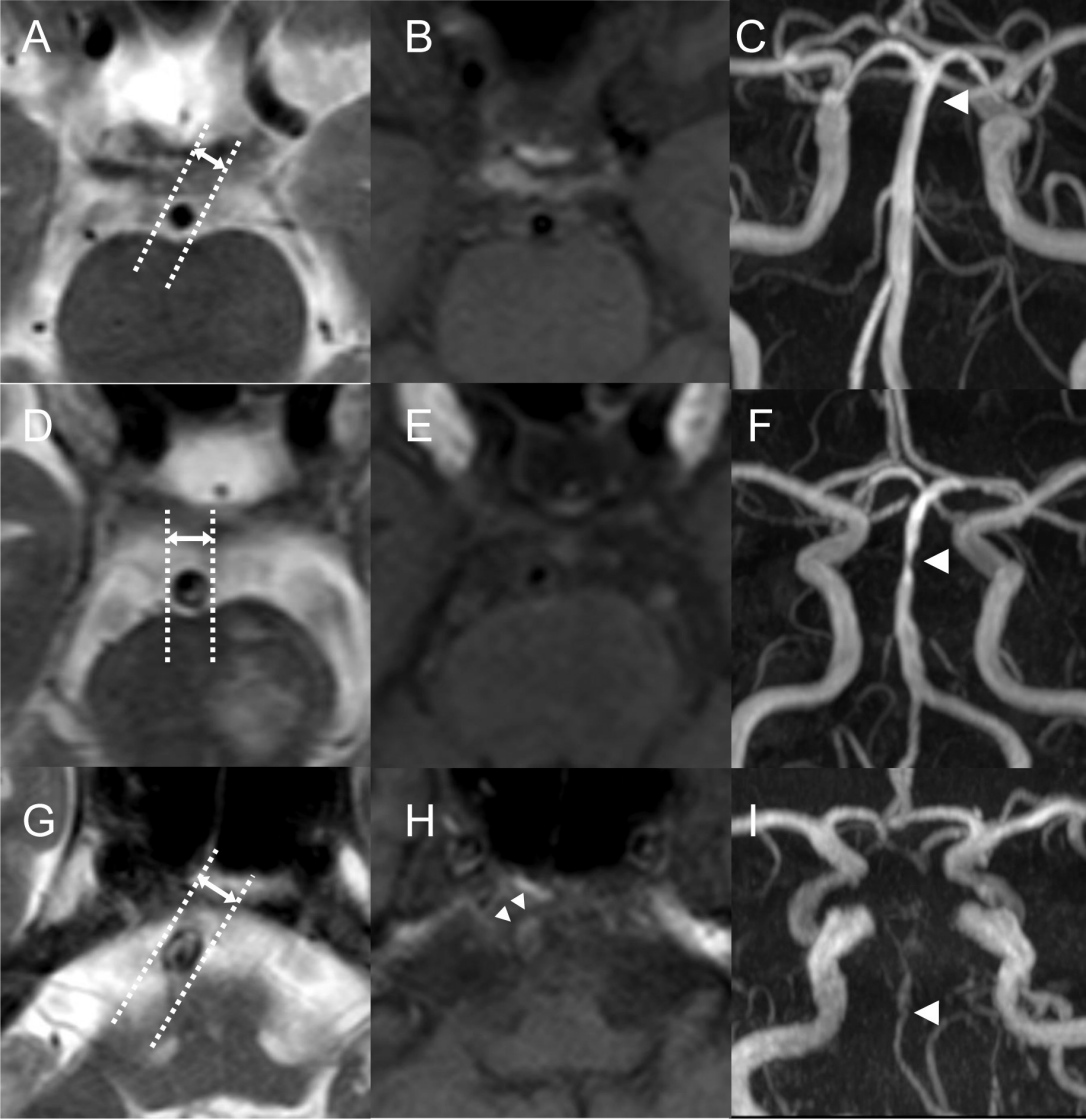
Representative basilar artery images. Left column, T2-weighted imaging; middle column, T1-weighted imaging; right column, MR angiogram. (A-C) Normal findings, with a diameter of 2.65 mm. (D-F) Eccentric T2 plaque sign (*) and increased diameter (4.51mm) were noted in a patient with 50%-69% stenosis. (G-I) T2 plaque sign (*), an increased diameter (5.4mm), and T1 hyperintensity within the plaque (H) in a patient with severe steno-occlusion of the basilar artery.
